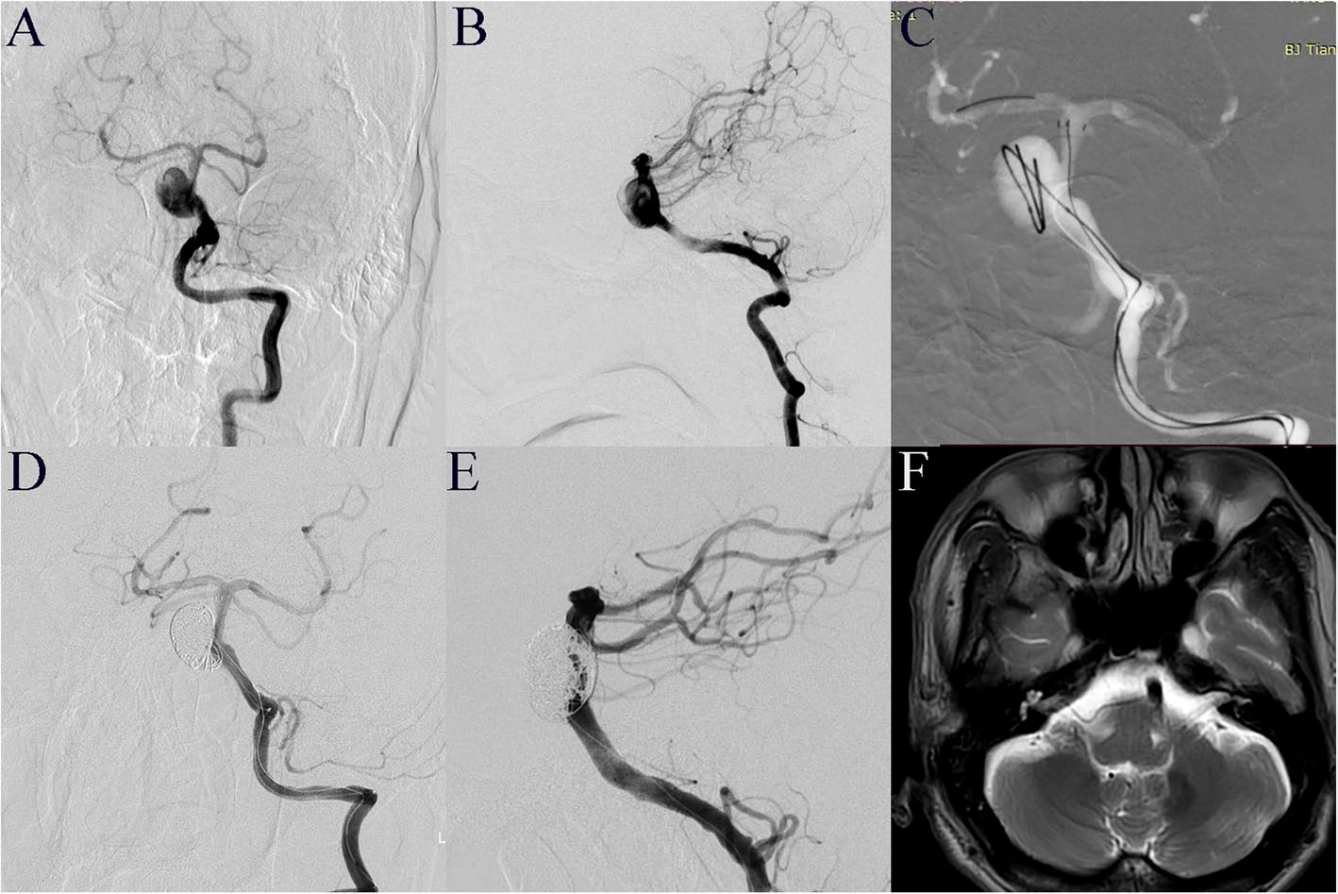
Infarction. A, B Left vertebral artery angiography demonstrates a basilar artery aneurysm in a 56-year-old man. C EP2 stent-assisted coiling was performed. D Angiography immediately after the operation showed complete aneurysm embolization and patency of the parent artery. F Magnetic resonance imaging obtained because of right upper arm weakness on the second day after the operation showed new infarction
